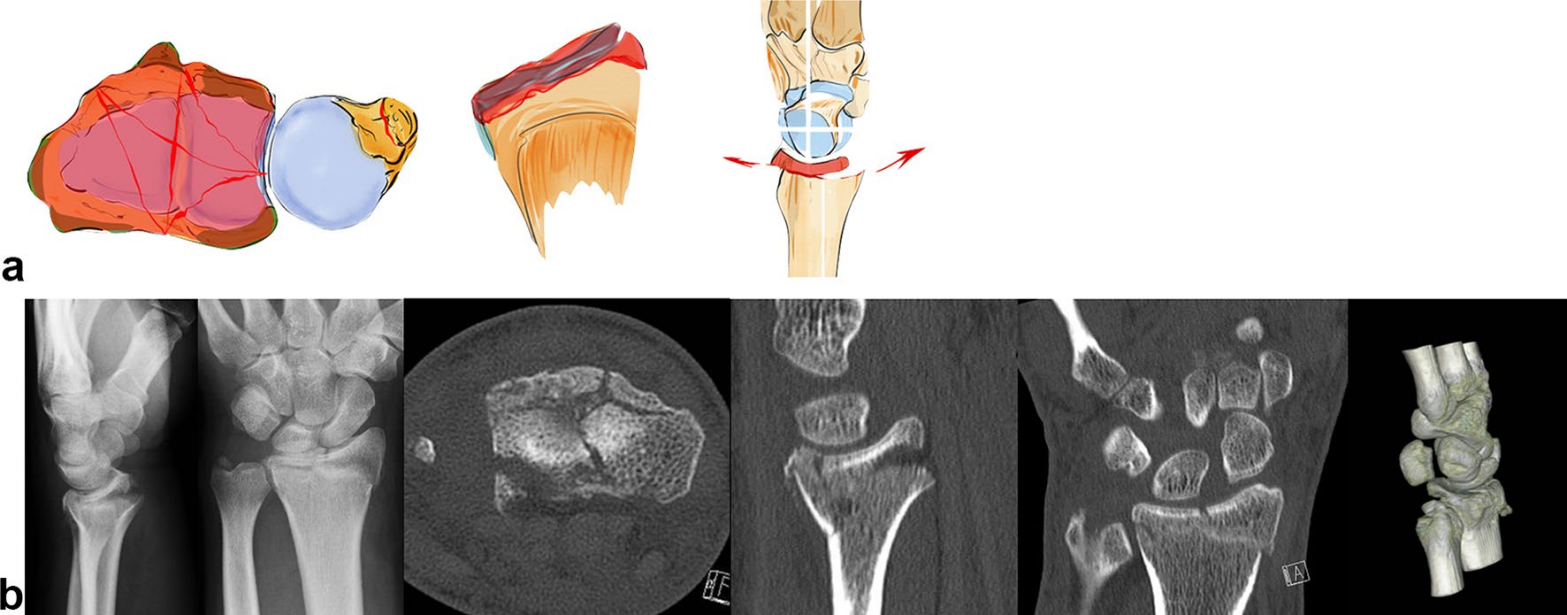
As can be seen on the CT scans, these distal shear fractures cause the fragments to float on the shaft of the radius like ice splinters. These are bony avulsions of all ligamentous insertions dorsal and palmar. Central shear fragments are without any ligamentous insertions. Since the fragments are extremely thin, it is difficult to grasp them and stabilize them sufficiently (a + b)
